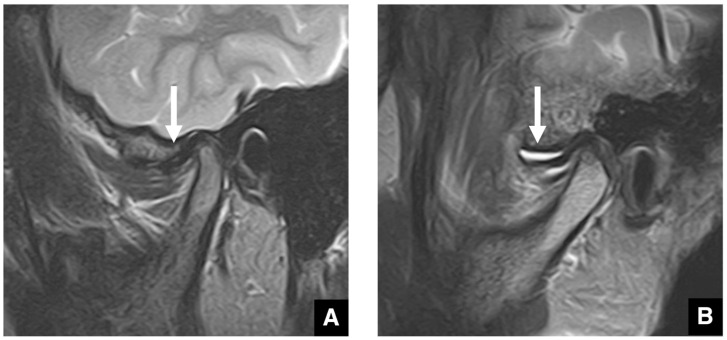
Comparison of a normal temporomandibular joint and a joint with TMJ effusion. In closed-mouth T2-weighted MR images (TR 4000 ms; TE 108 ms; slice thickness 3.0 mm), (A) no areas of high signal indicative of fluid are observed in the joint space (arrow), and (B) a high-signal area indicating TMJ effusion is observed in the superior joint space (arrow). Permission has been obtained to publish all figures.
